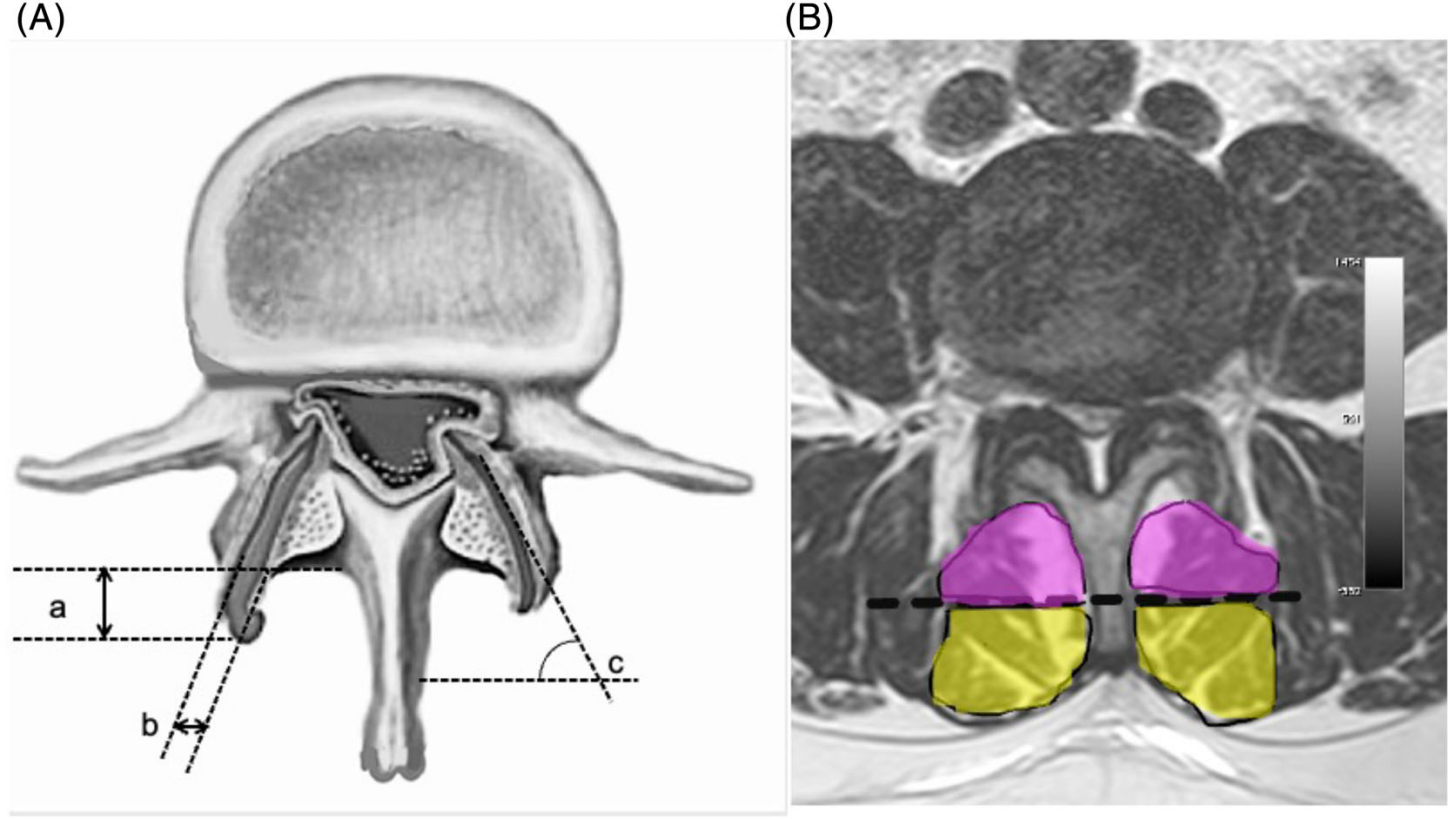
(A) Facet joint morphological features measurements: Facet overhang (a), facet joint opening (b), and facet angle (c). (B) Muscle measurements. Magnetic resonance image, T2 weighted axial cut showing the margins of the multifidus. (Deep fibers of the multifidus muscle were defined as short interlaminar fibers located deep to the midpoint between the base and tip of the spinous process. Deep part of the multifidus tCSA is highlighted in purple and superficial part tCSA is highlighted in yellow
